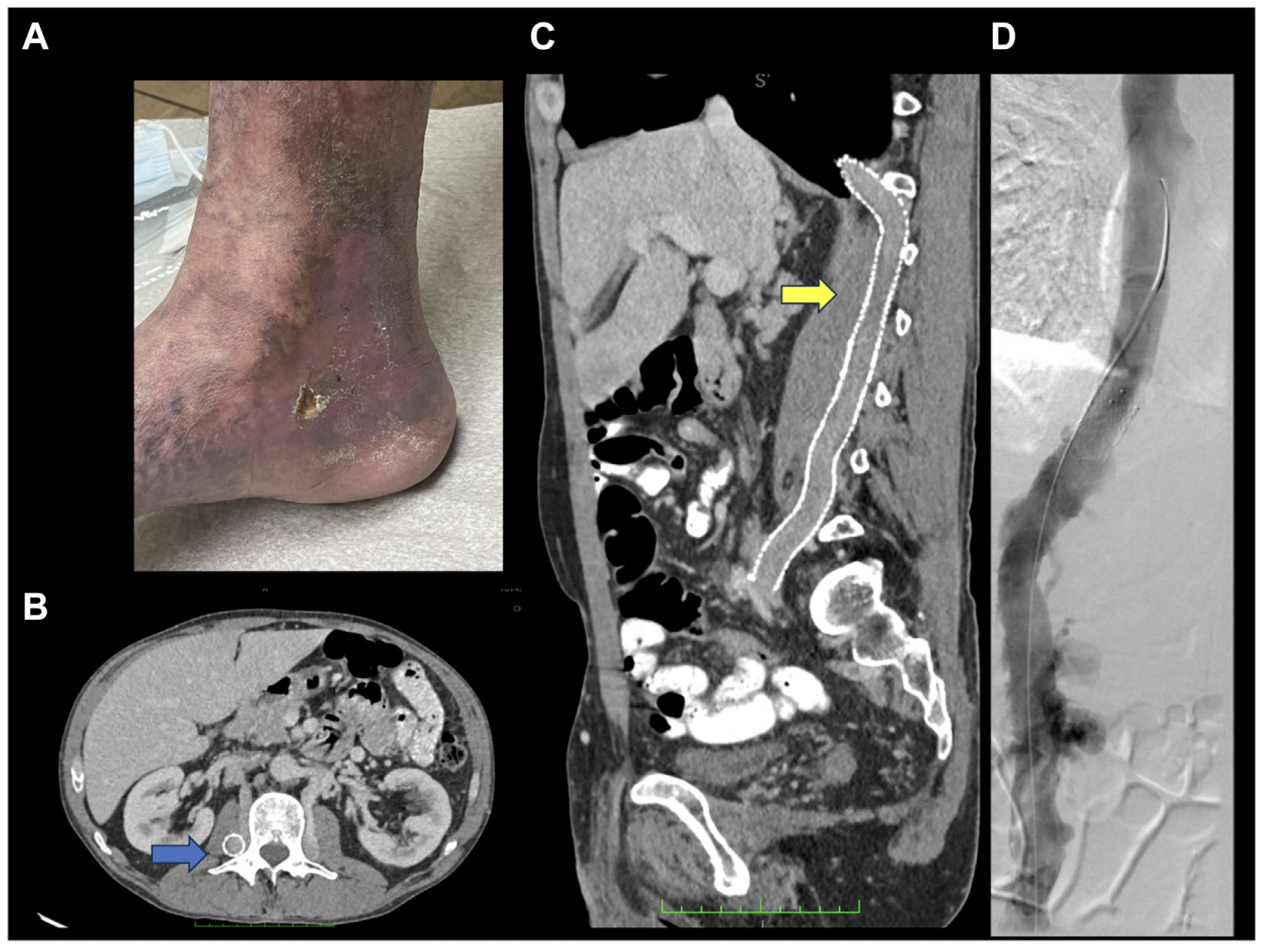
A, Photograph showing a venous ulcer at the right medial malleolus. B, Axial computed tomography scan showing azygous stent (*blue arrow*). C, Coronal computed tomography scan showing azygos stent draining into suprahepatic vena cava (*yellow arrow*). D, Venogram of the azygos vein after stenting.
